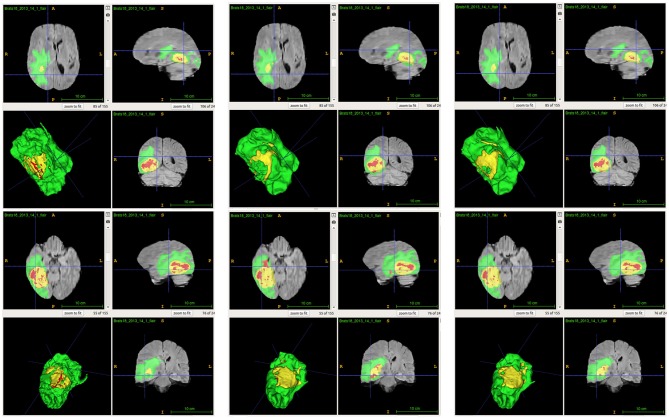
Examples of predictions from ensemble with different inputs. Top row shows the predictions from ensemble of DeepMedics, and bottom row shows the predictions from ensemble of 3D U-Nets (from left to right: ground-truth lesions, prediction from ensemble, and prediction from ensemble with additional brain parcellation masks.) Red: enhancing tumor, yellow: necrosis & non-enhancing tumor, and green: edema. ITK-SNAP (Fedorov et al., 2012) is used to visualize the MR images and lesion masks.
